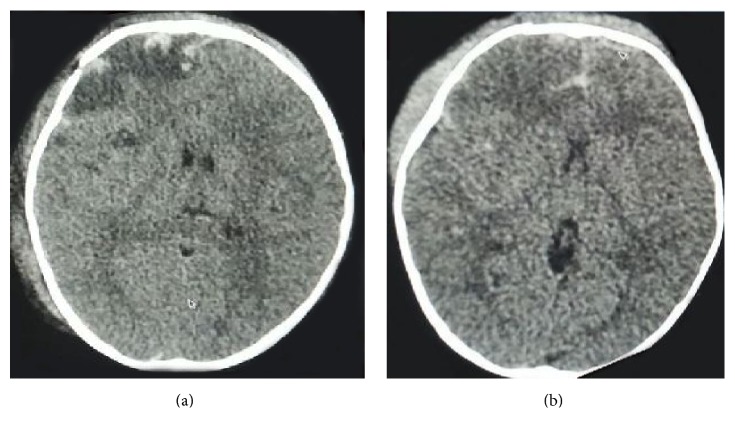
Computed tomography of head showing fracture of right frontal bone and subdural hematoma and extradural hematoma with contusion and subarachnoid hemorrhage.
